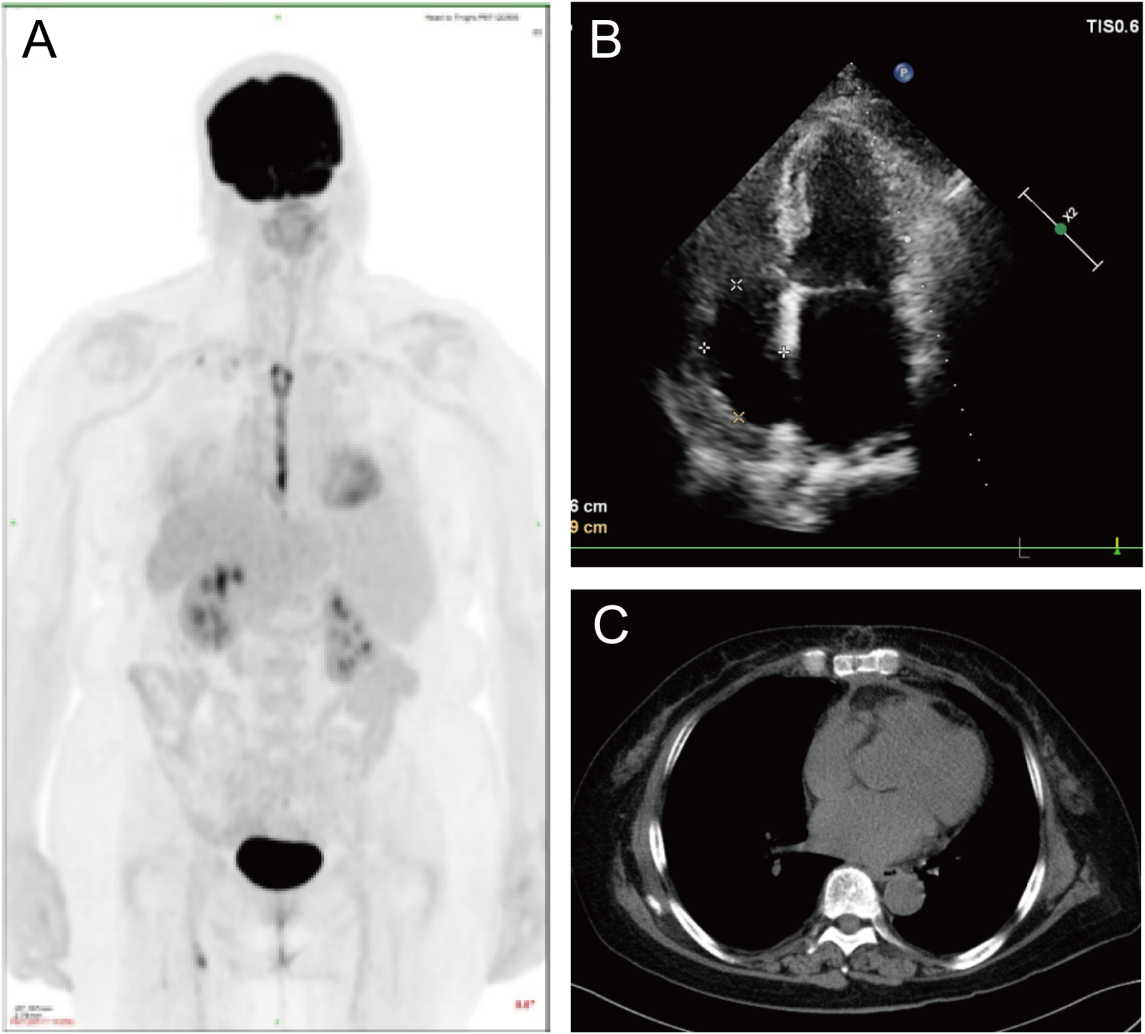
Follow-up imaging. PET-MR (A), echocardiography (B), and chest CT (C), demonstrated no evidence of recurrence or residual disease.
